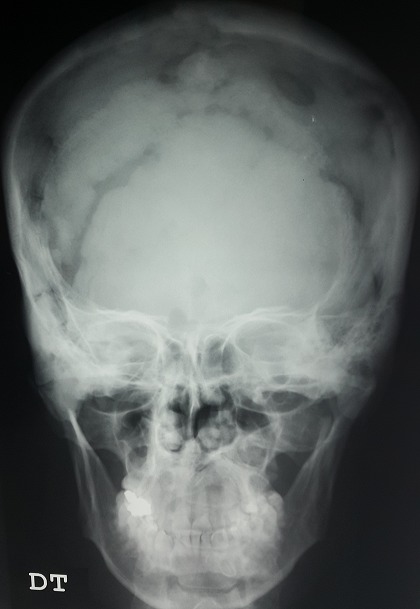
Radiographie standard crane de face montrant de multiples lésions lacunaires osseuses
